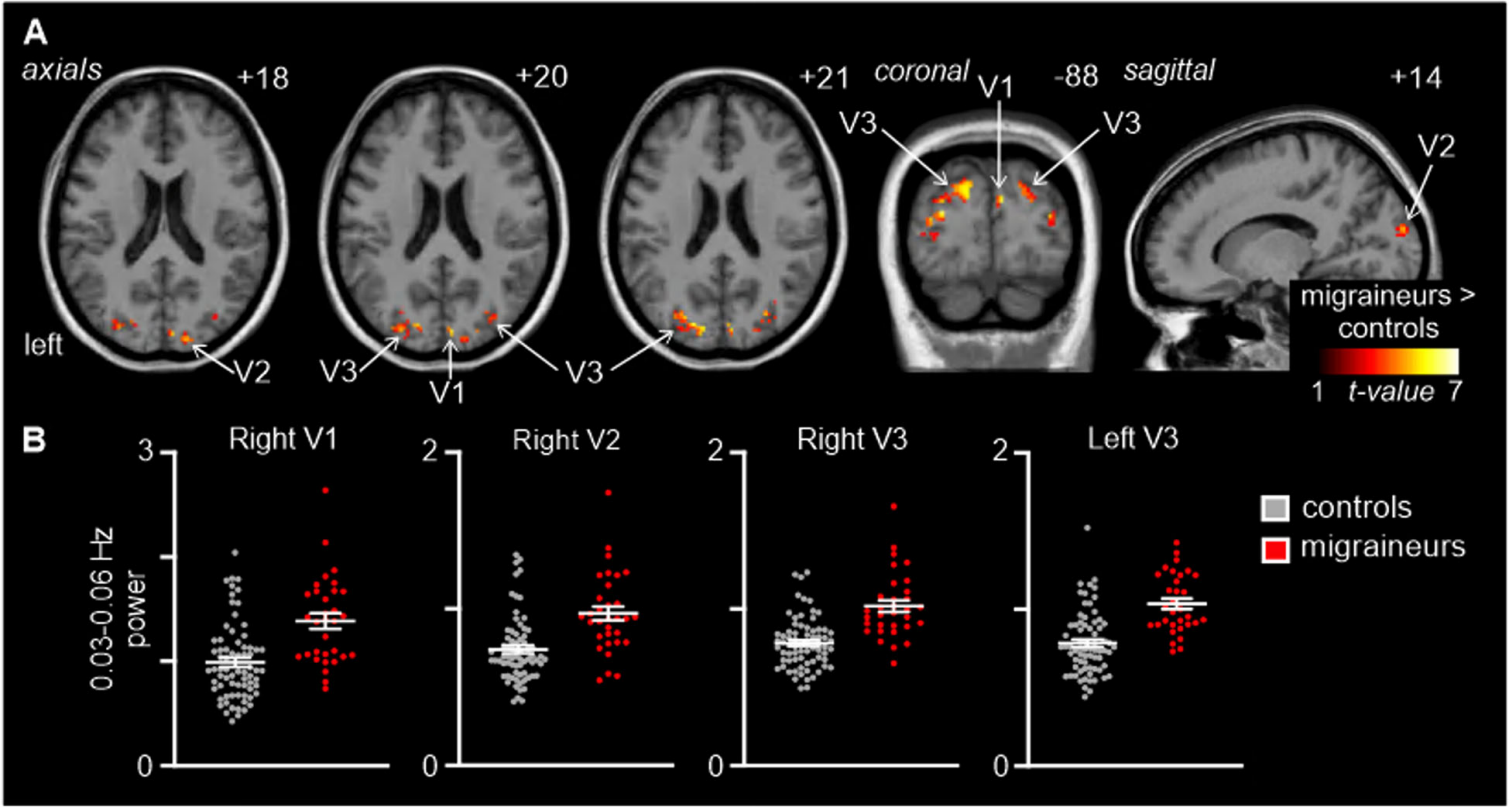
A. Significantly higher resting infra-slow (0.03–0.06 Hz) power in migraineurs (*n* = 32) compared with controls (*n* = 71) in the occipital lobe (random effects, *p* < 0.05, corrected). Significant clusters are overlaid onto a mean T1-weighted anatomical image and the location of each axial, coronal and sagittal slice in Montreal Neurological Institute space indicated at the top right of each slice. B. Plots of individual subject and mean (± standard error of mean) power in four significant clusters. *V1: primary visual cortex; V2: secondary visual cortex; V3: third visual complex*
